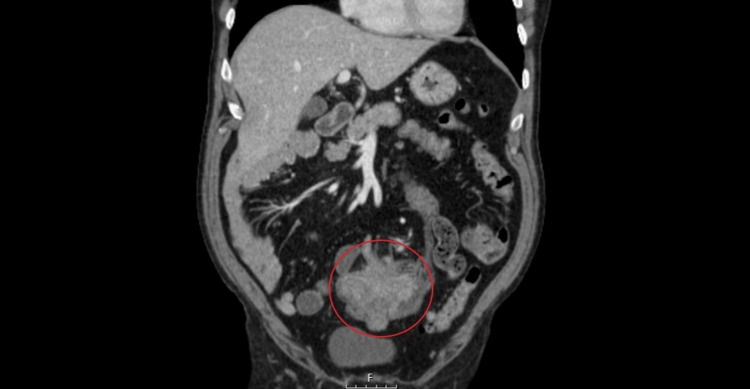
Coronal contrast-enhanced CT scan showing a caecum tumor located in the midline (red circle).
